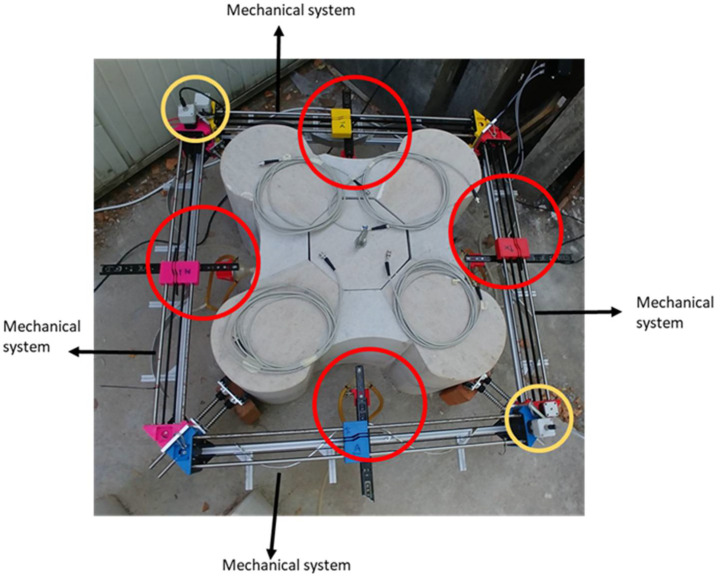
Ultrasonic tomography system developed. Red circle: coupling system with transducer + motion cart and yellow circle: motors.
